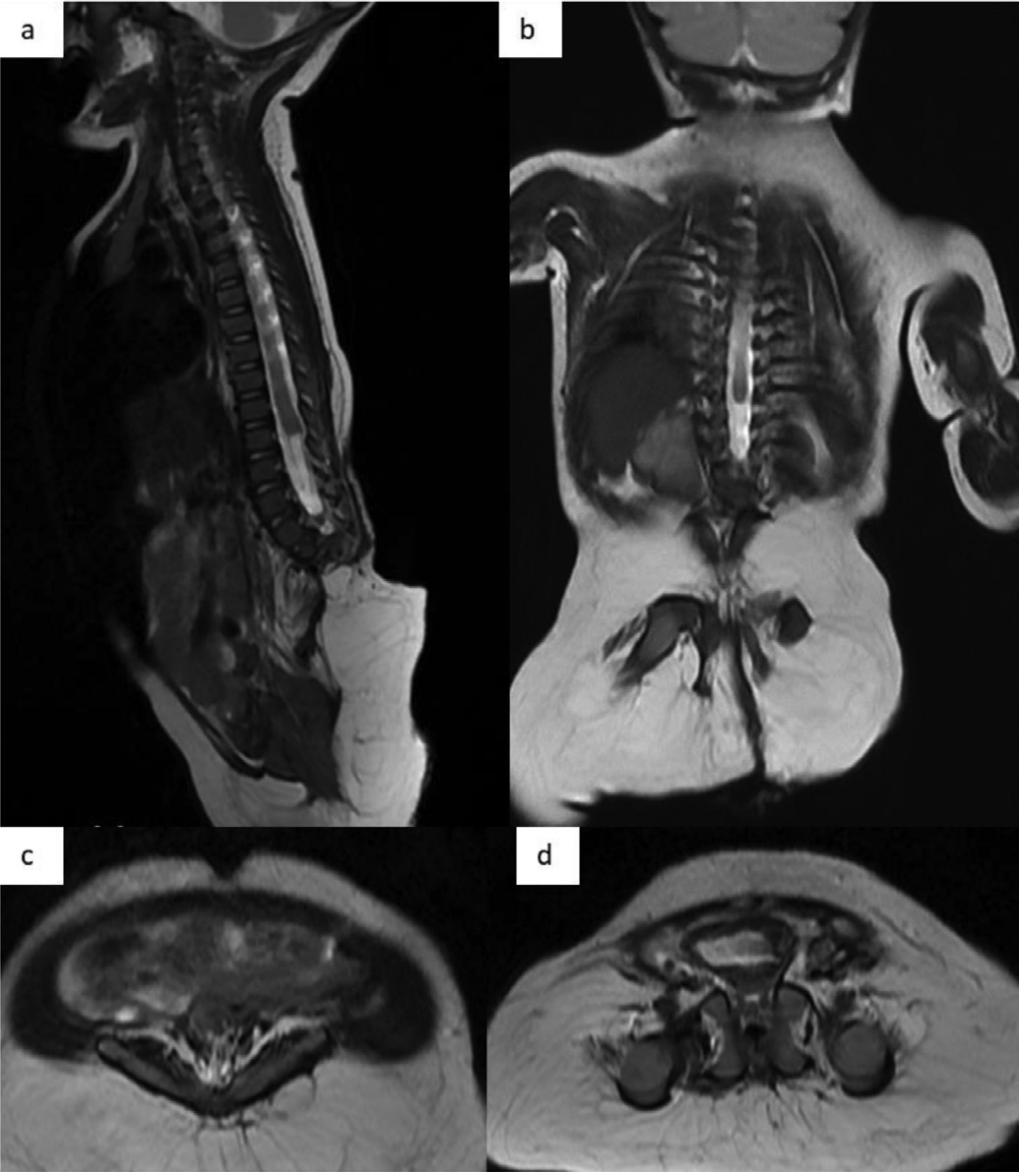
(A, B) An MRI of whole spine at T2 sequences reveals termination of the spinal cord at level of the thoracic 9 vertebra (T9). There is defect at L1 and agenesis of L2-L5 vertebrae, which is replaced with fatty structure. Malformation revealed is identified to be a total sacral agenesis and iliac wing agenesis, consistent with either Type IV (Renshaw) or Type I (Pang) Caudal Regression Syndrome (C, D) An axial view MRI of the pelvic and sacral fusion displays the bladder protruding into the anterior abdominal wall.
